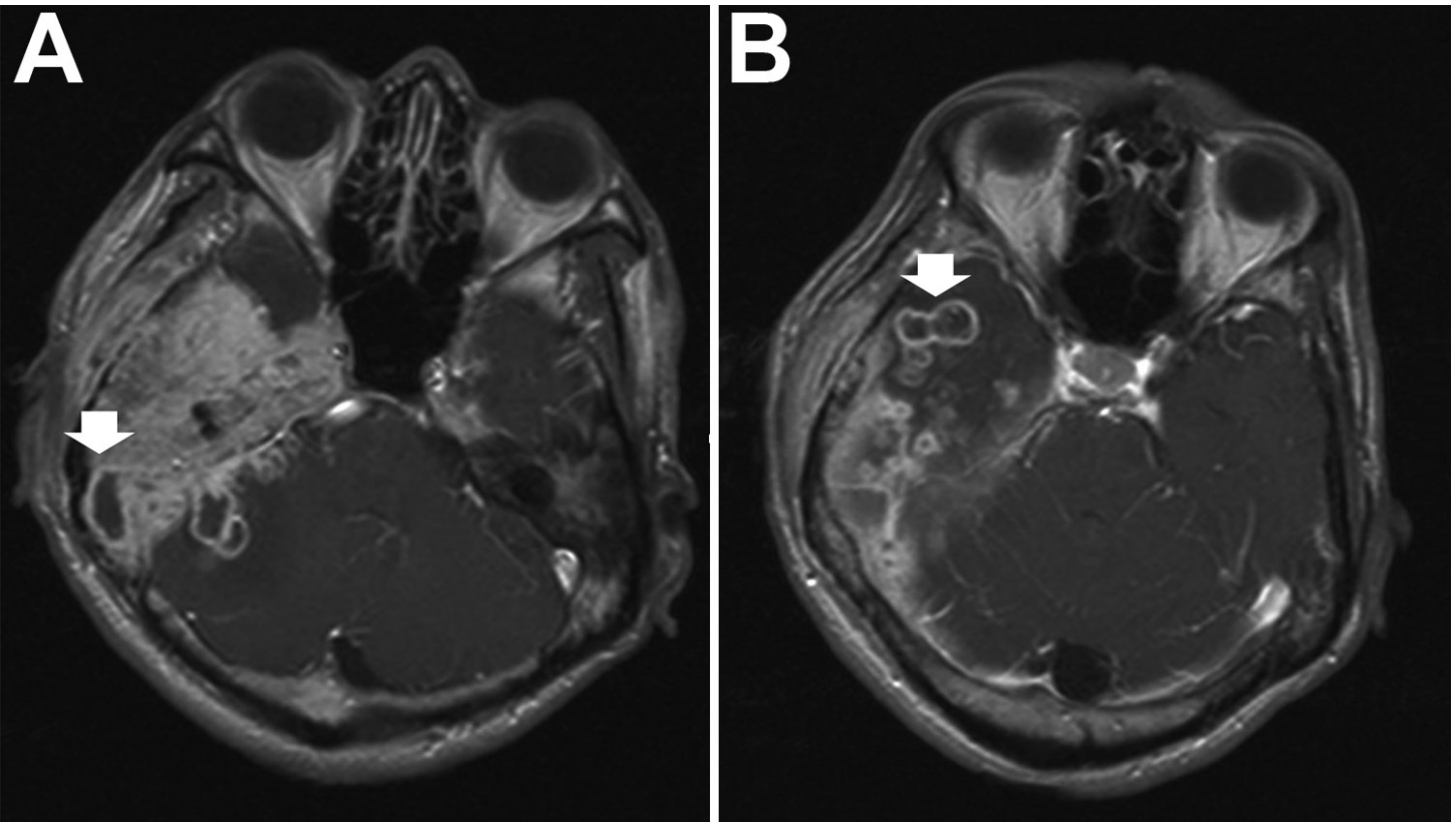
Brain computed tomography scan images for a patient with central nervous system infection caused by *Mycobacterium abscessus* subsp. *bolletii*. Arrows indicate abnormal nodular pachymeningeal thickening and leptomeningeal and intraparenchymal extension with multiple rim-enhancing lesions in the right cerebellum (A) and right temporal lobe (B), indicating cerebral abscesses.
